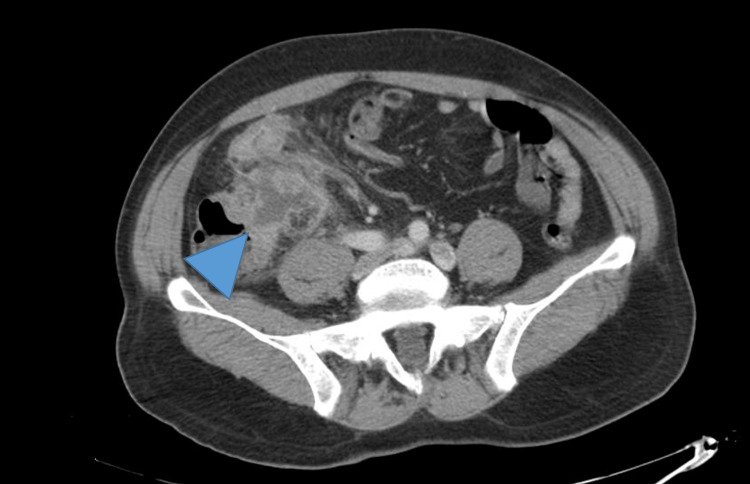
Periappendicular abscess. CT scan axial view showing periappendicular abscess (arrowhead).
